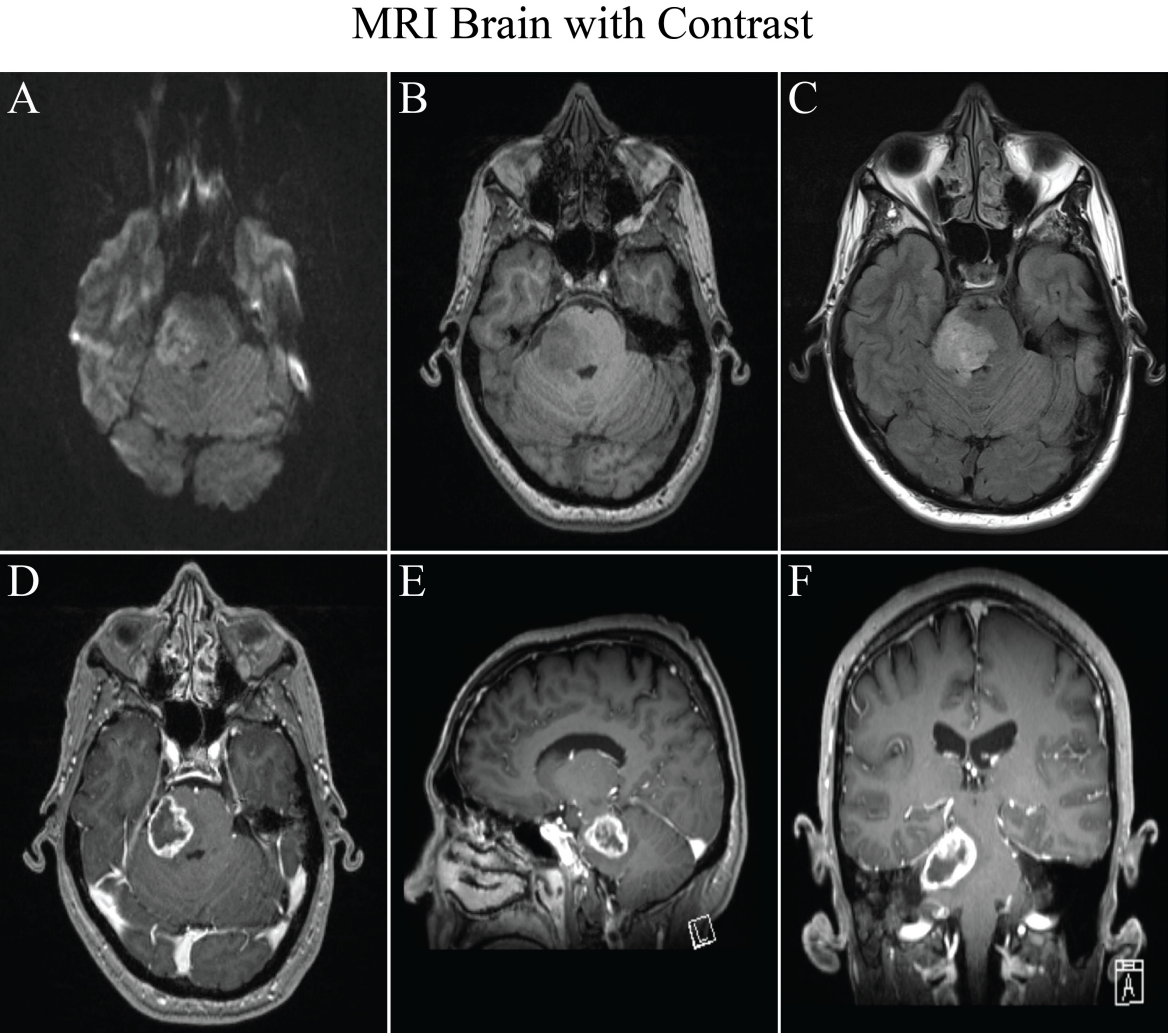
MRI brain with contrast at diagnosis A. Diffusion weighted imaging displaying no restricted diffusion. B. T1 pre-contrast. C. T2 FLAIR. D. Axial T1 post-contrast. E. Sagittal post-contrast. F. Coronal post-contrast.
